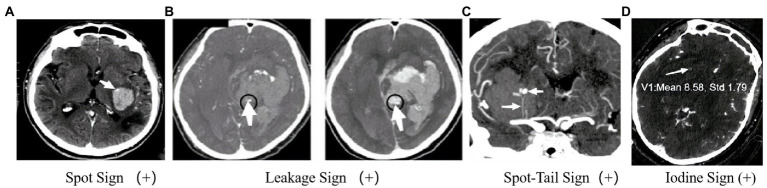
Imagination markers on CTA. (A) Enhanced focus within a hematoma indicates contrast agent extravasation and reveals the site of vessel rupture (5); (B) In the CTA phase, an enhanced focus within the hematoma is observed, and further observation in the delayed CTA phase can identify an increase in CT value within a specific region of interest. A larger enhanced focus may indicate persistent bleeding and HE (6). (C) The presence of an intrahematoma striate artery and an enhanced focus within the hematoma may suggest an arterial rupture and pinpoint the location of bleeding (7). (D) In GSI, an internal focus with an IC >7.82100 μg/ml indicates a positive IoS (8).
